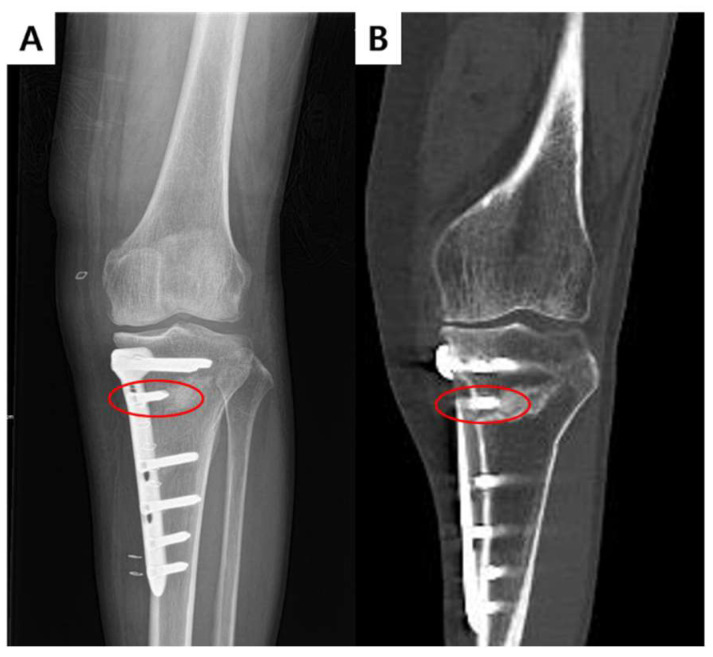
(A) Postoperative X-ray shows the D-hole screw of the conventional anatomic plate violating the osteotomy plane. (B) Postoperative 3D computed tomography scan showing D-hole violation.
